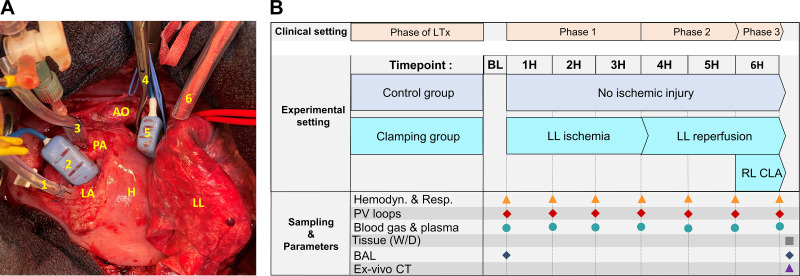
A*: open chest experimental setup demonstrating the instrumentation. *B*: clinical lung transplantation (LTx) setting vs. experimental protocol: overview of sampling and parameter recording. AO, aorta; H, heart; LA, left atrium; LL – left lung; PA, pulmonary artery.1—LA pressure catheter; 2—PA flow probe; 3—PA pressure catheter; 4—vascular clamp on left PA and left main bronchus; 5—left PA flow probe; 6—vessel loop around Right PA enabling its closure during 6H; BL, baseline; 1H, 1 hour; 2H, 2 hours; 3H, 3 hours; 4H, 4 hours; 5H, 5 hours; 6H, 6 hours; RL CLA, right PA clamping; Hemodyn., hemodynamic parameters, Resp., respiratory parameters; PV, pressure-volume; *W*/*D*, wet-to-dry ratio; BAL, bronchoalveolar lavage; CT, computed tomography.
